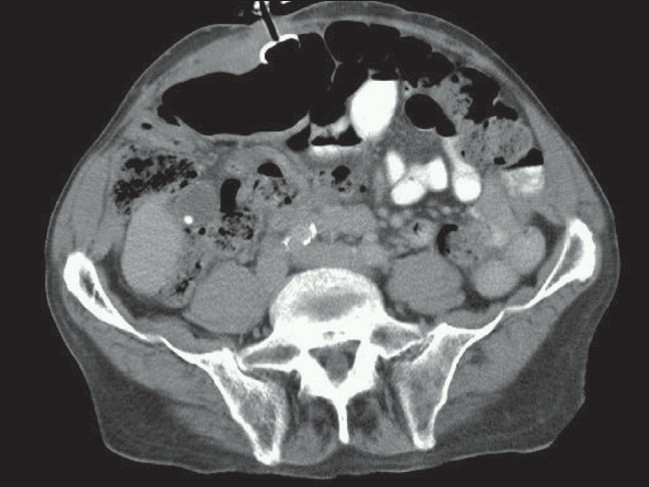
Post Op CT scan checking the position of the tubes and excluding major complications
